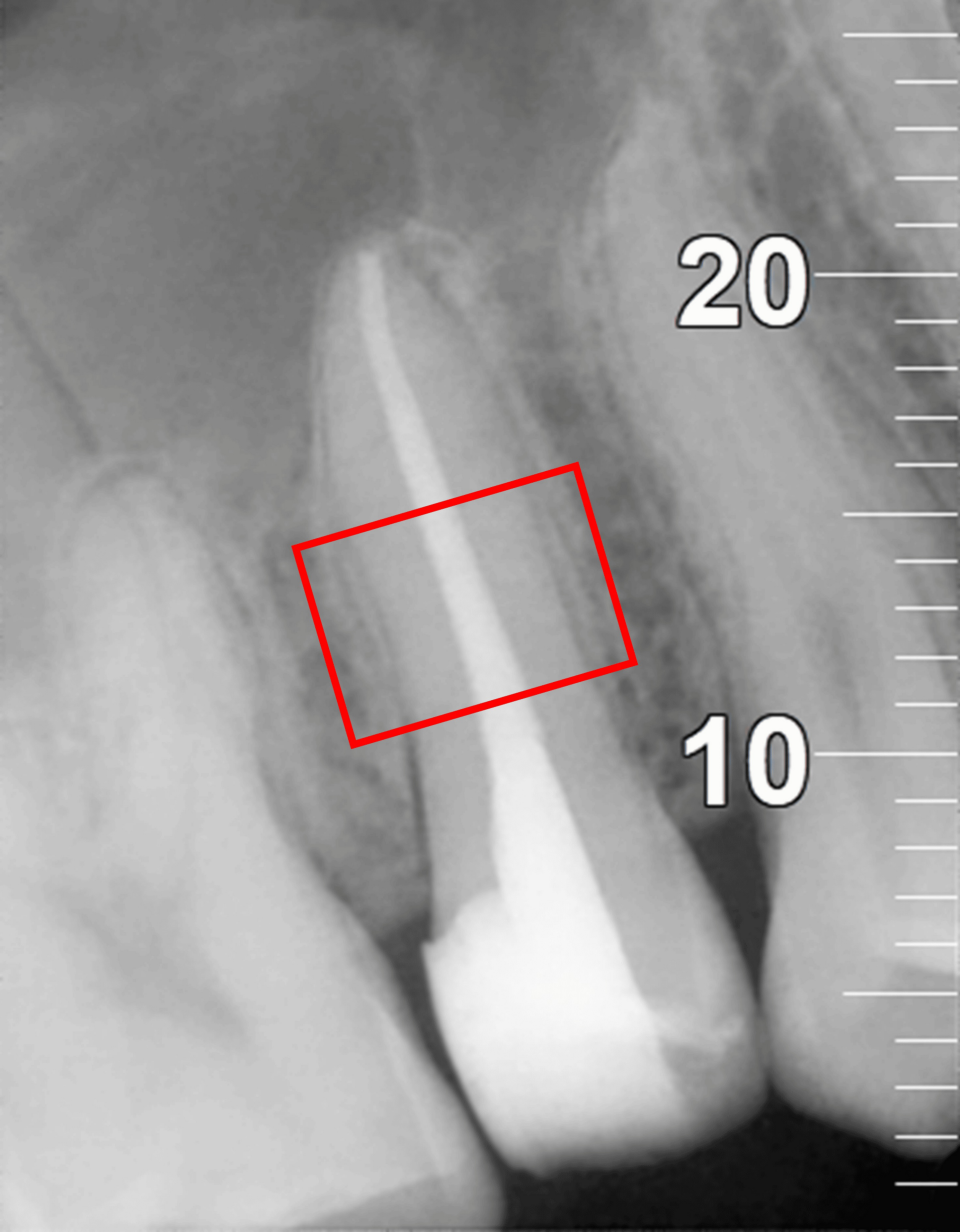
Region of interest (ROI), i.e., middle third of the root canal system marked for analysis on the radiograph to calculate the brightness percentage.
